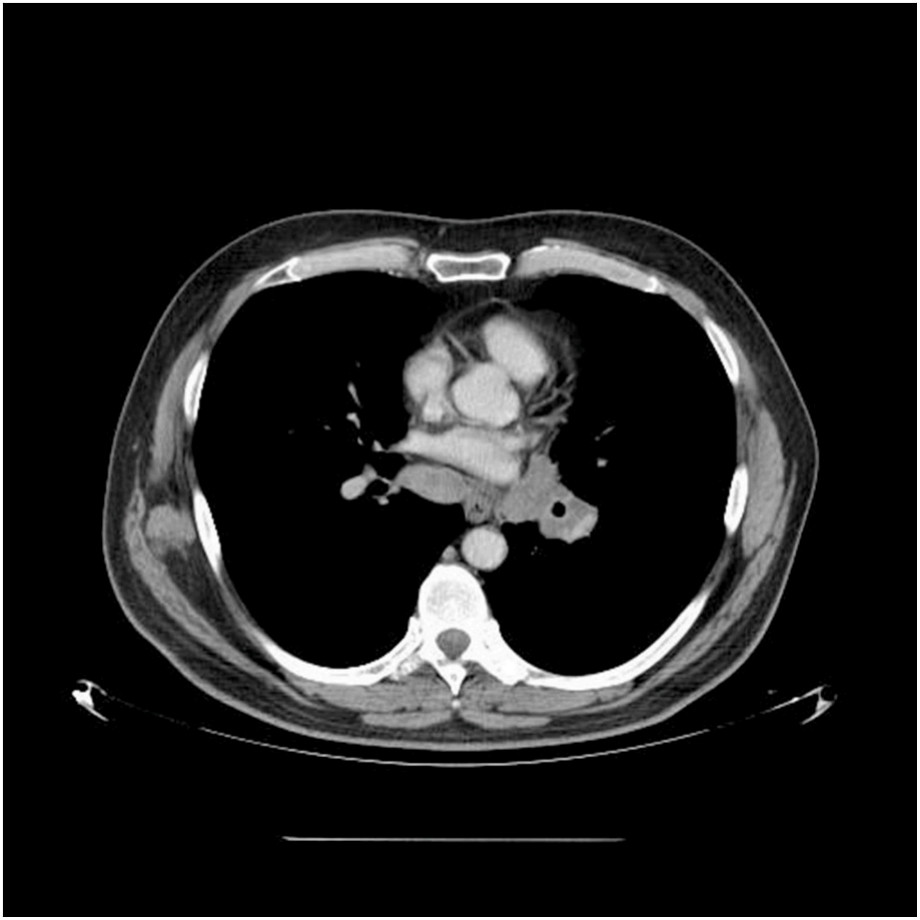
A chest CT scan showed a left hilar tumor with metastases in the mediastinal lymph nodes.
